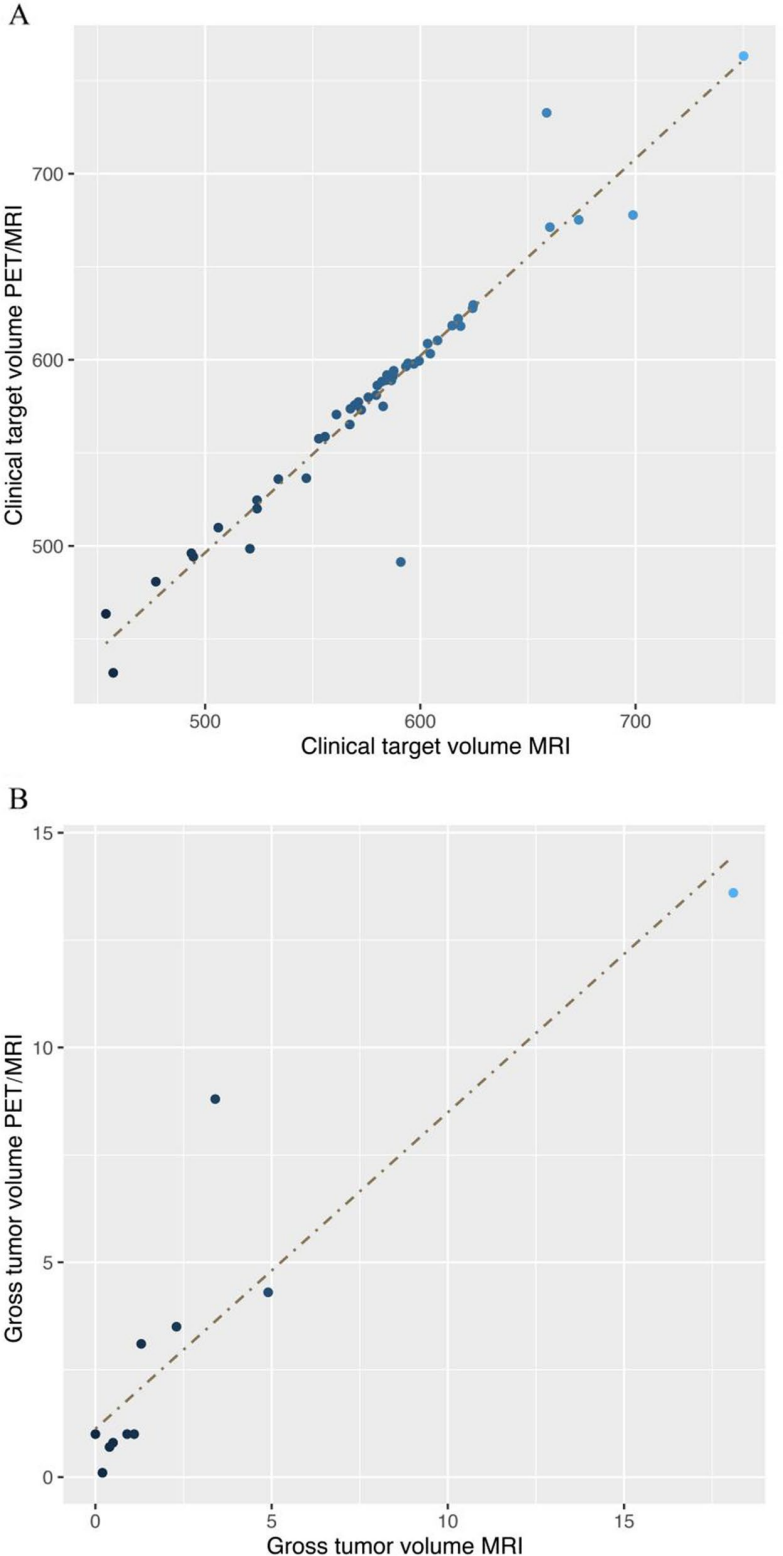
PSMA PET/MRI-CTV versus MRI-CTV (A); PSMA PET/MRI-GTVn versus MRI-GTVn (B)
